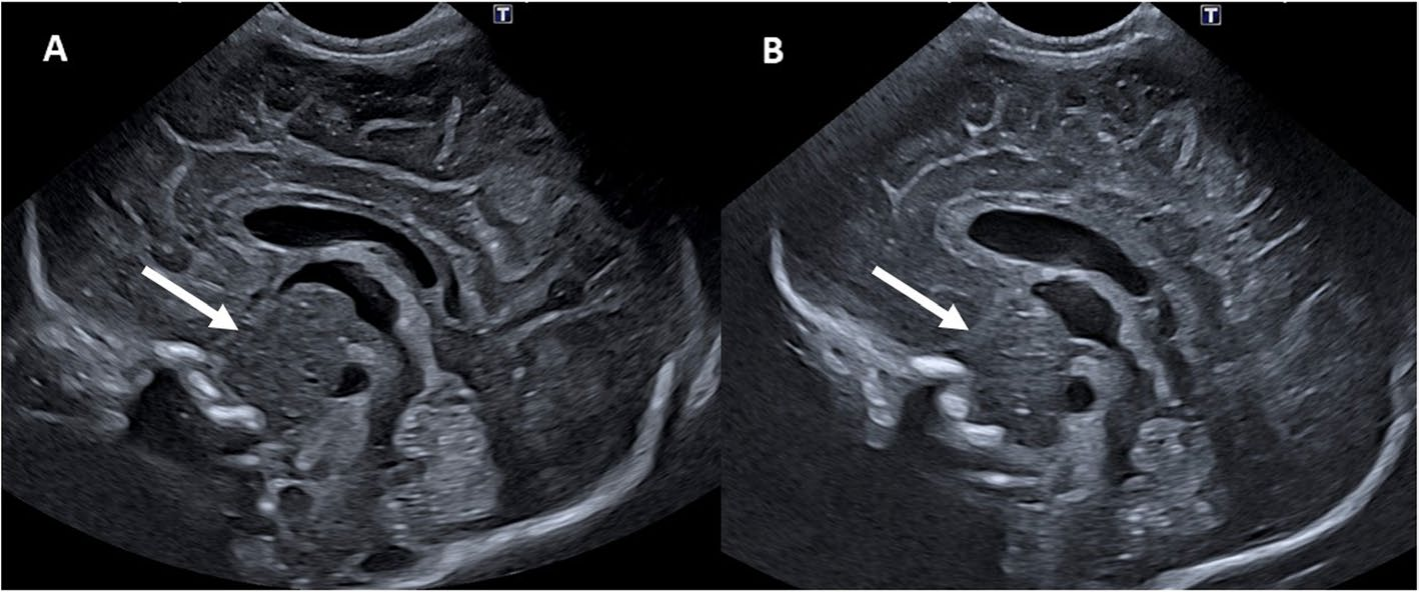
Mid-sagittal ultrasound scan through the anterior fontanel: A Twin A; B Twin B. White arrows indicate a round mass-like lesion in the suprasellar region, anterior to the brainstem, isoechoic to the surrounding parenchyma. The third ventricle’s floor is superiorly displaced, with a patent Sylvian aqueduct
